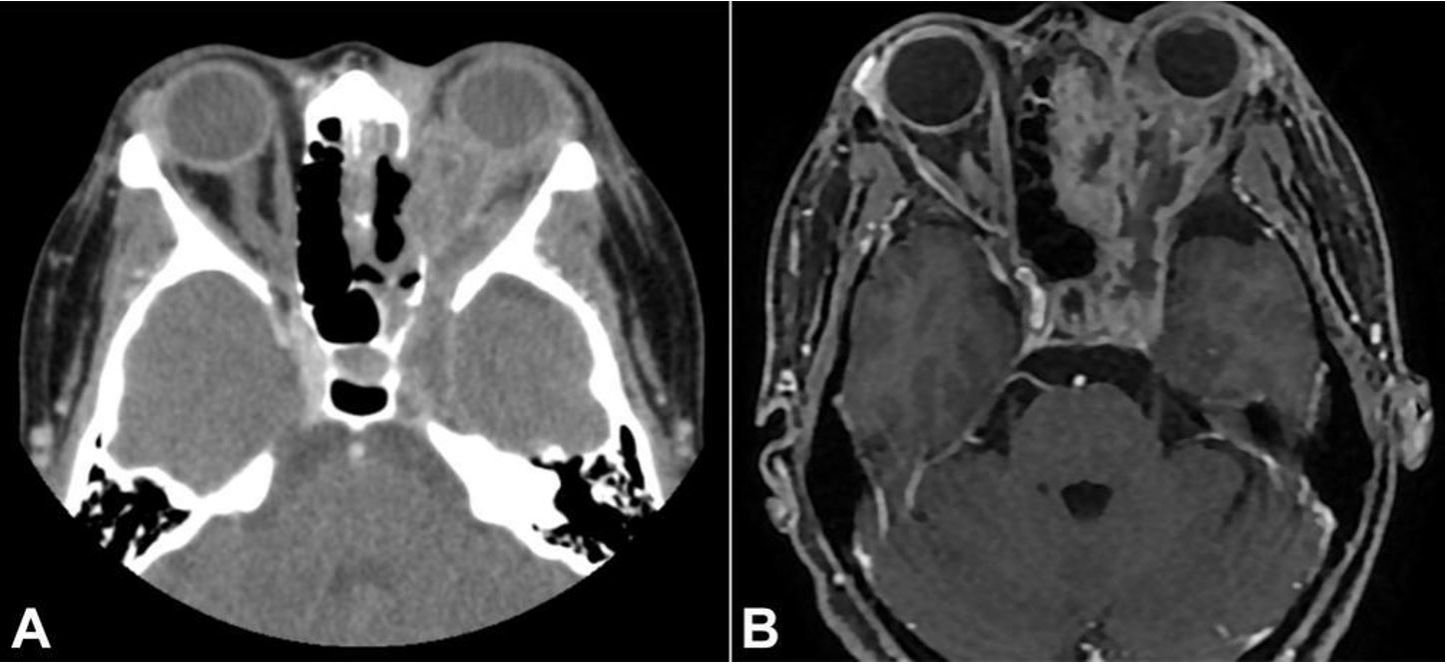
Computed tomography and magnetic resonance imaging of the orbital region: A – diffuse obliteration of the orbital fat on the left by tissue that involves the extraconal and intraconal compartments, more accentuated in the posterior portion of the orbit; B – diffuse thickening and peripheral enhancement of the sheath/optic nerve complex, suggesting direct infiltration by the fungal material.
